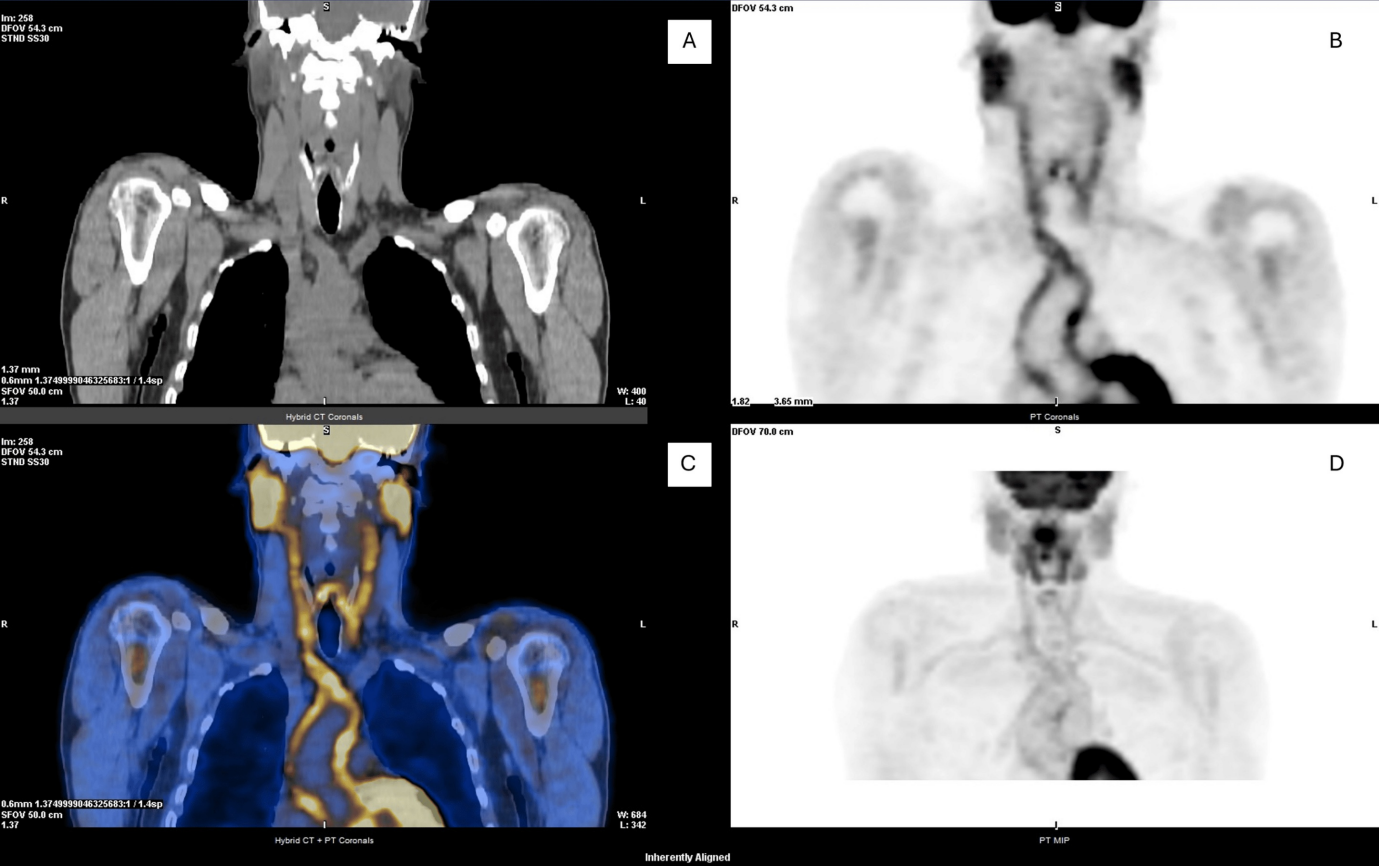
18F-FDG PET/CT imaging. Corresponding CT (A), PET (B), fused PET/CT (C), and PET MIP (D) images showing increased FDG uptake in the thoracic aorta, brachiocephalic trunk, and bilateral carotid arteries, consistent with active large-vessel vasculitis. 18F-FDG, 18F-fluorodeoxyglucose; CT, computed tomography; MIP, maximum intensity projection; PET, positron emission tomography
